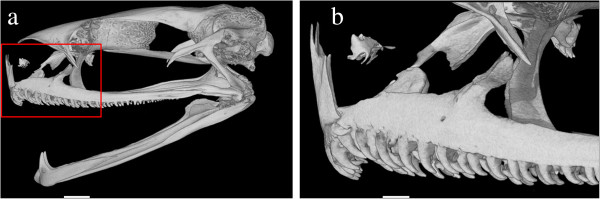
CT-scan of *Odontobatrachus *skull. Computer tomographic scan in lateral view of *Odontobatrachus natator* skull (ZMB 78203) a: lateral view of left side, lower jaw virtually rotated to open the mouth, red square defines close-up shown in b; scale bar = 1.5 mm; b: close up of the anterior part of the maxilla; scale bar = 0.5 mm. The images highlighting the tusk-like odontoids on the lower mandible as well as curved and pointed pre- and maxillary teeth; skull virtually isolated from the remaining skeleton.
